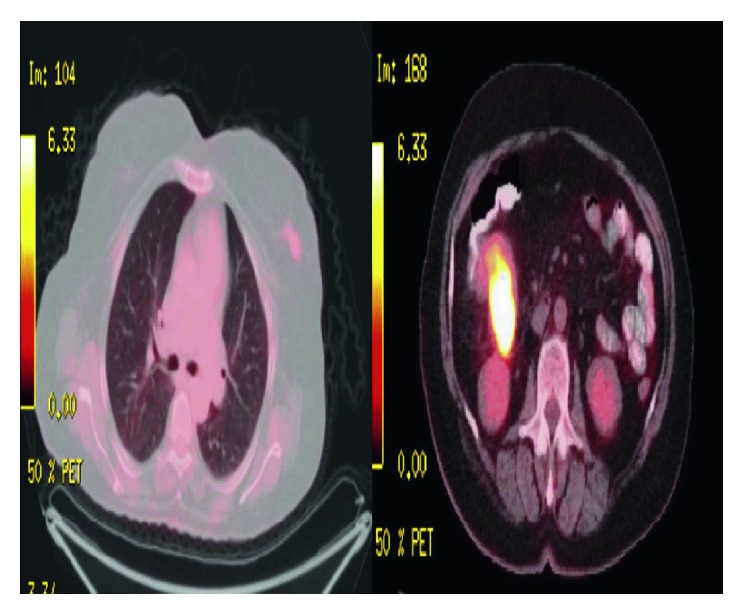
PET-CT showing increased FDG uptake.
